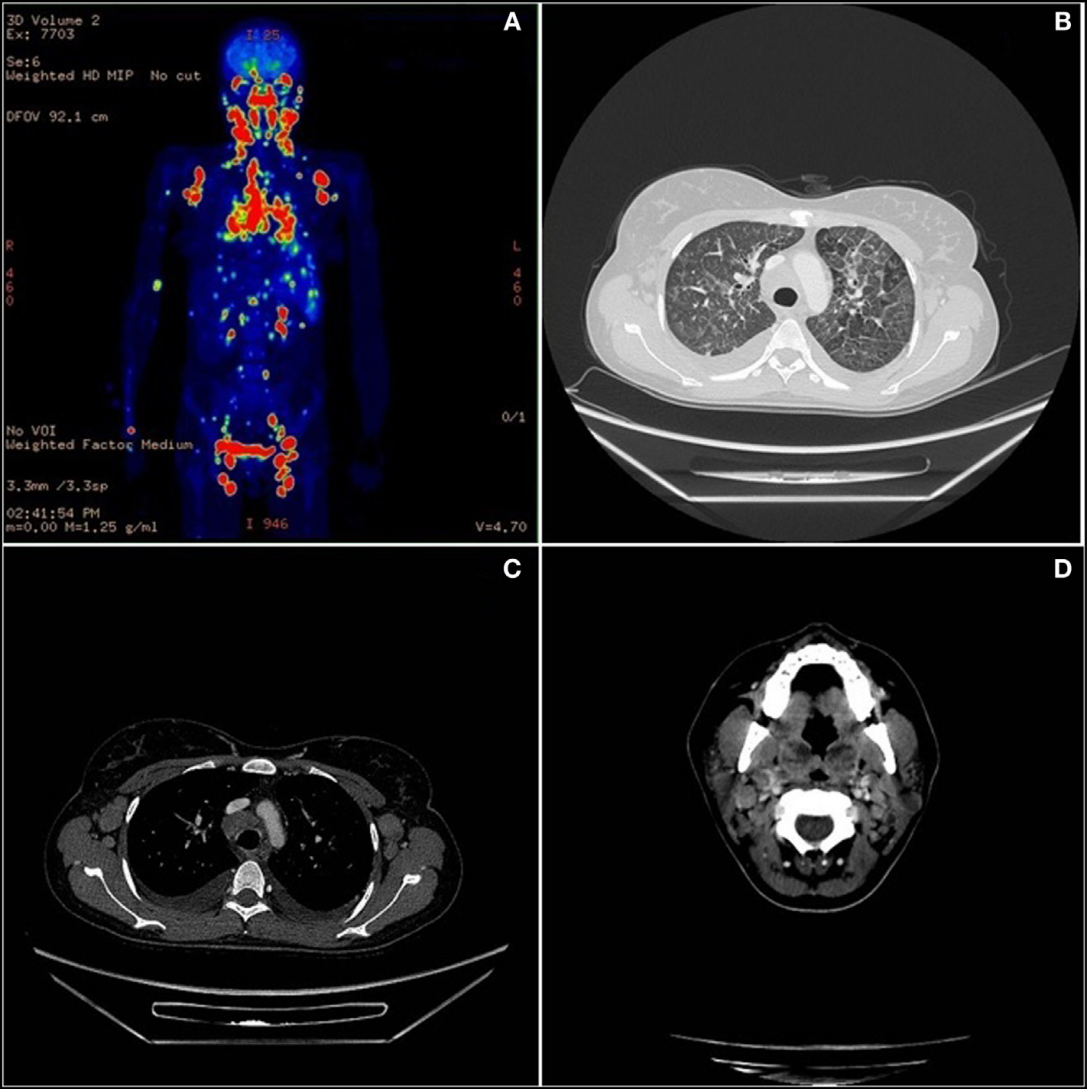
Total body positron emission tomography (PET)–computed tomography (CT). (A) Diffuse lymph nodes involvement in PET. (B) Lymphoma lung interstitial reticular thickening in CT scan. (C) Axillary and mediastinum enlargement lymph nodes in CT scan. (D) Neoplastic involvement of neck nodes and palatine tonsils in CT scan.
